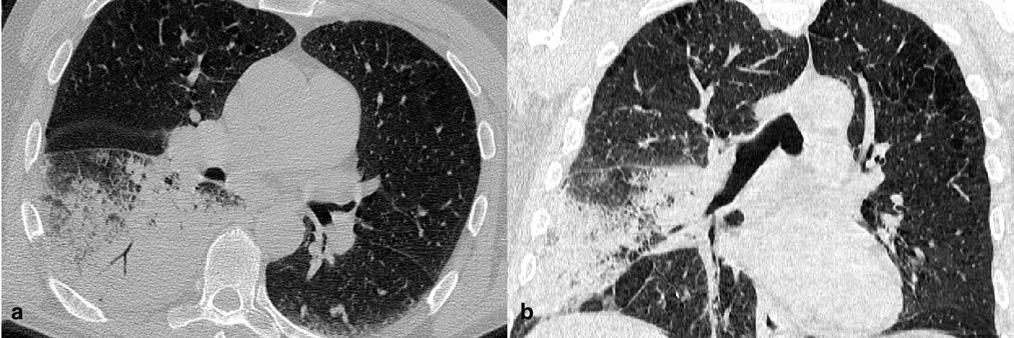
A 57-year-old COVID-19 patient (δ variant) presenting fever and dry cough for 3 days. Axial (a) and coronal (b) reformatted CT images showed pneumonia with isolated lobar consolidation in the right lower lobe.
